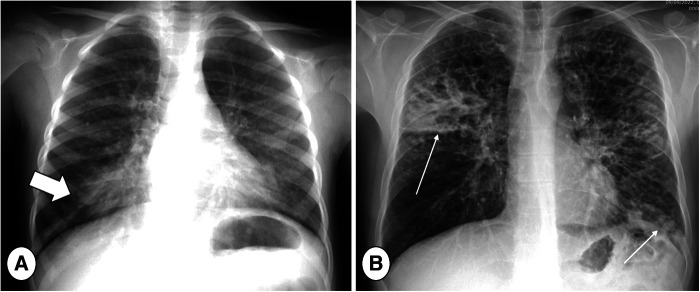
Posterior-Anterior (PA) chest radiograph in mild (A) and severe (B) cystic fibrosis lung disease during respiratory tract exacerbation. Note large consolidation in the right lung base in image A indicating respiratory tract exacerbation (thick arrow) and bilateral consolidations in the right upper lobe and left lung base (B, thin arrows).
